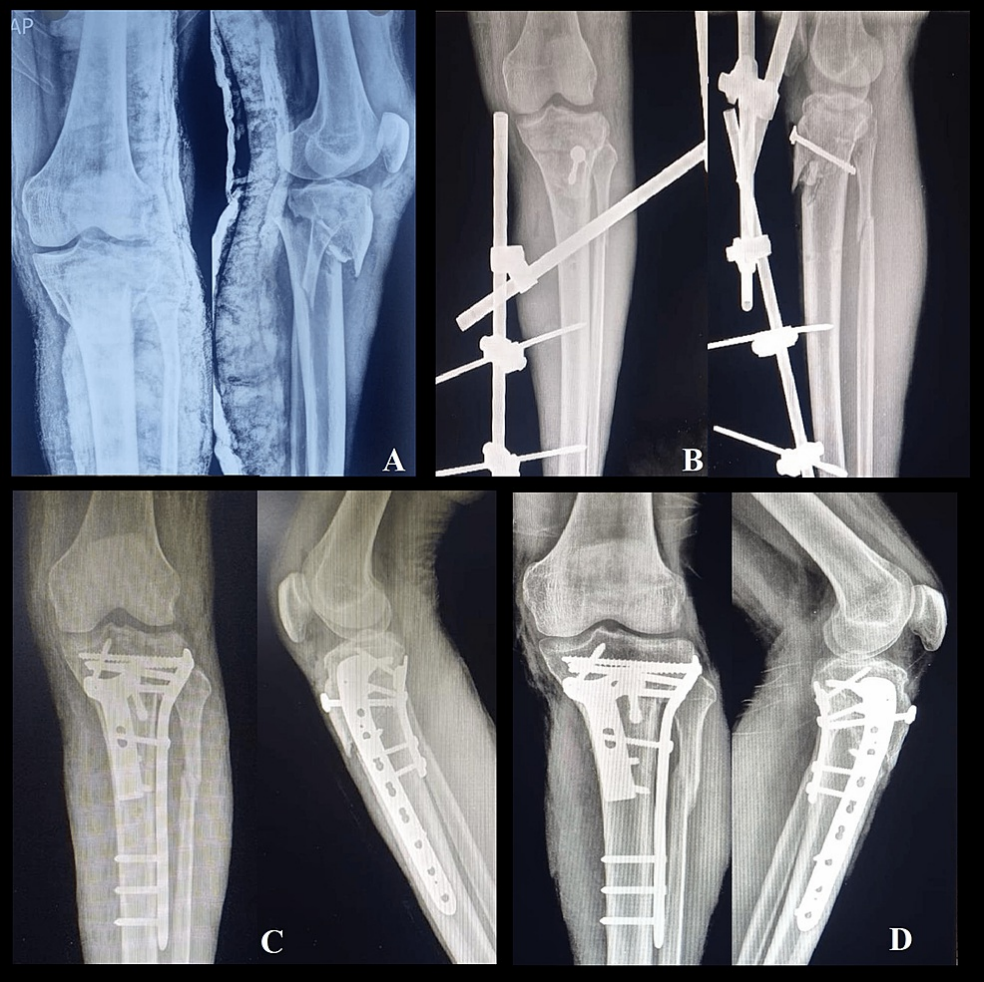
Serial follow-up radiographs of left side complex tibial plateau fracture in a 35-year-old male managed with staged procedures due to soft tissue compromise at presentation. A: Preoperative radiographs of the sustained fracture managed with primary splintage using a plaster-of-Paris slab.
